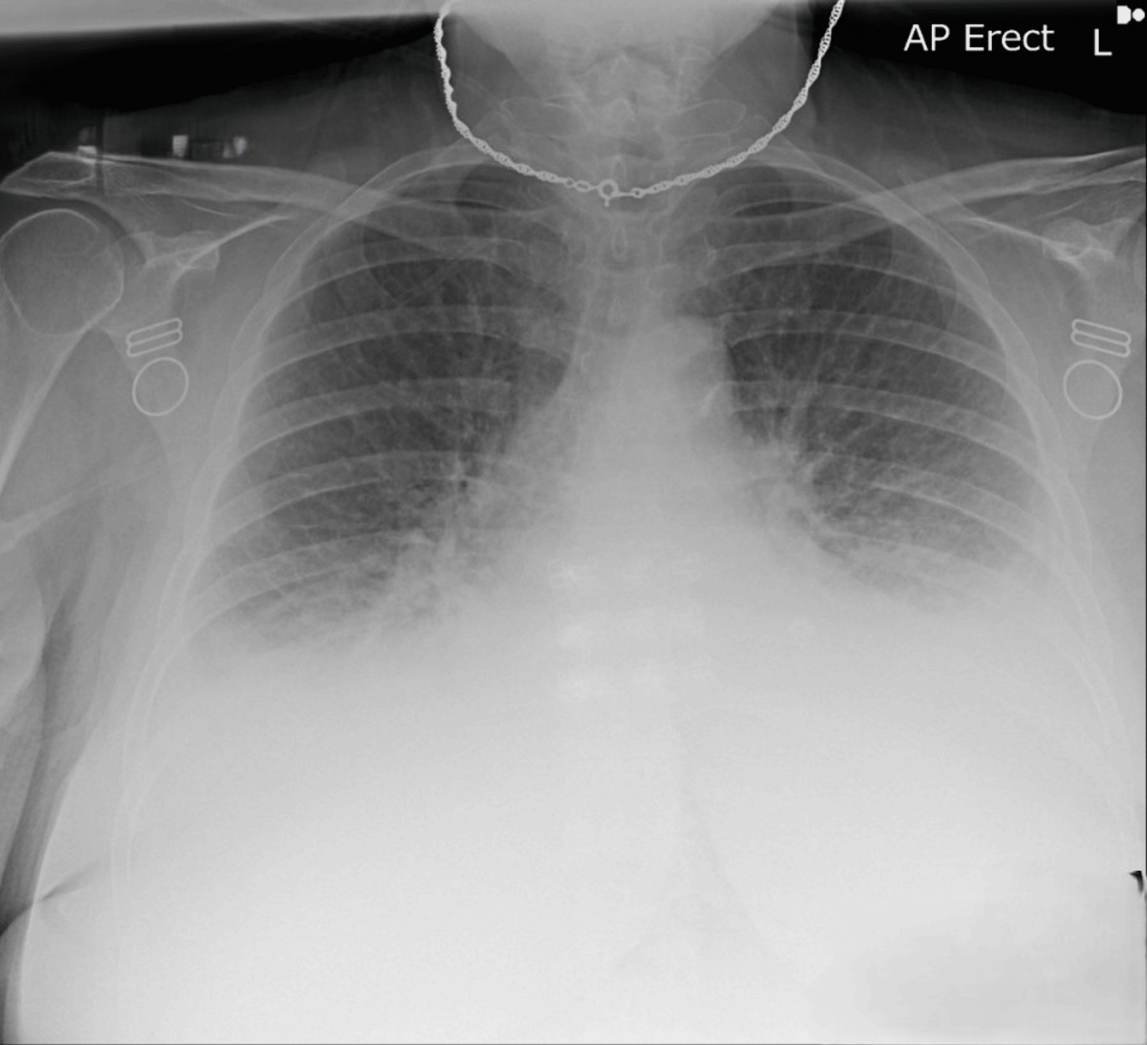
Chest X-ray showed features of heart failure, including bilateral pleural effusion, congested hila, and pulmonary edema.
